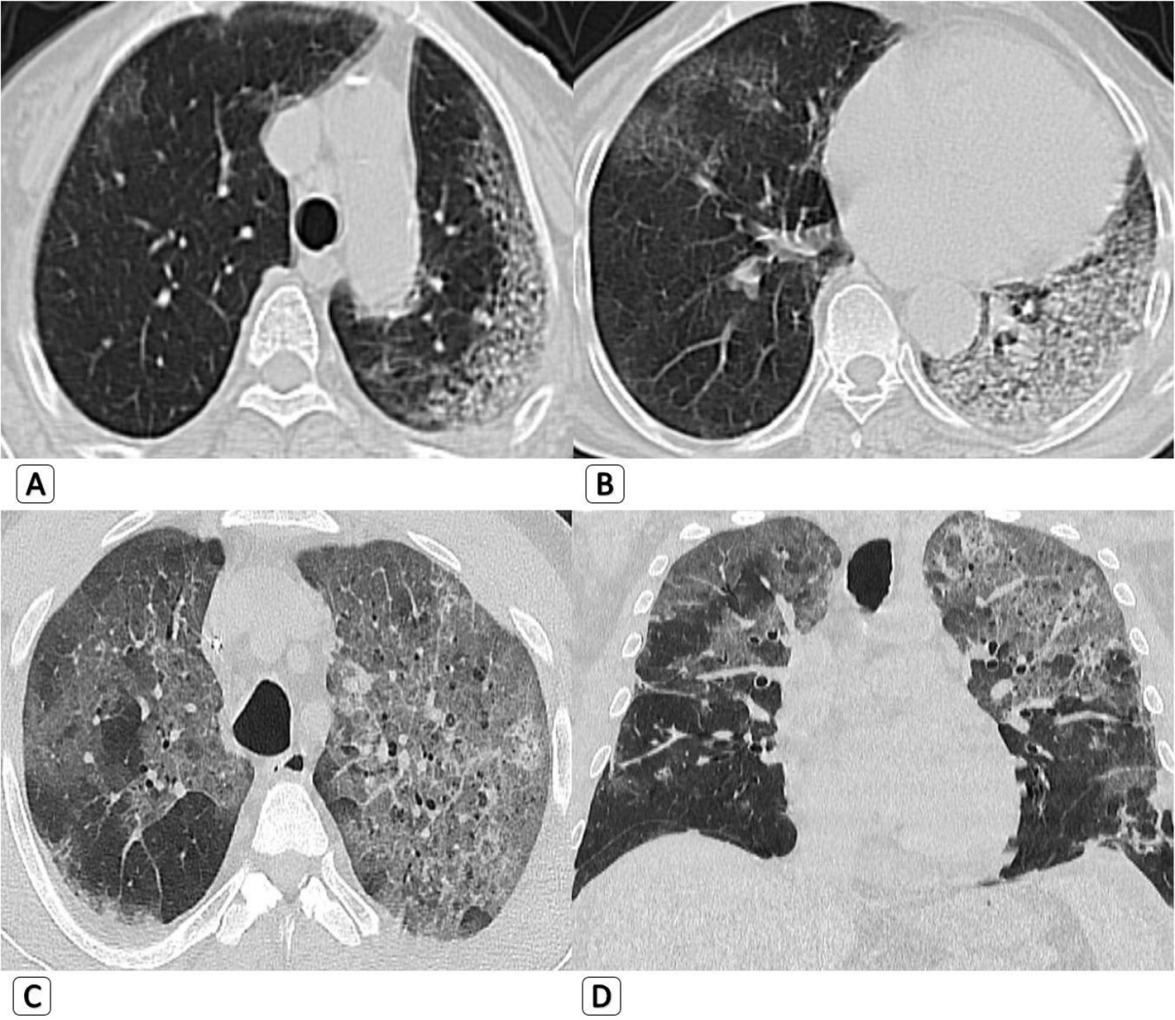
Severe cases complicated by diffuse alveolar damage (DAD), which cannot differentiate COVID19 from H1N1. a, b 52-year-old male *COVID-19 patient* with axial HRCT chest lung window showing bilateral ground glass opacities, more extensive and dense on the left side showing “crazy paving pattern” and mixed with mild air filled cystic changes denoting “early DAD.” c, d 58-year-old male *H1N1 patient* with axial and coronal HRCT chest lung window showing bilateral, predominantly upper lobar, ground glass opacity showing “crazy paving pattern” and mixed with air dilled cystic changes denoting “DAD”
